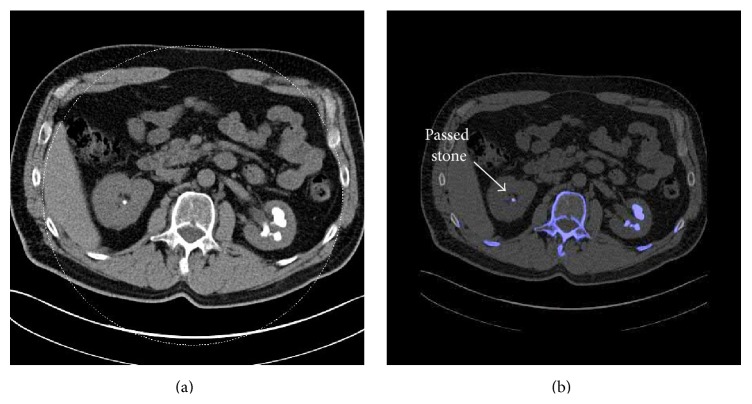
Conventional CT (a) and DECT (b) images showing a large staghorn stone and a small stone in the left and right kidneys, respectively. The standard DECT postprocessing algorithm colors all non-uric-acid stones blue. A follow-up scan confirmed the location of the passed stone.
